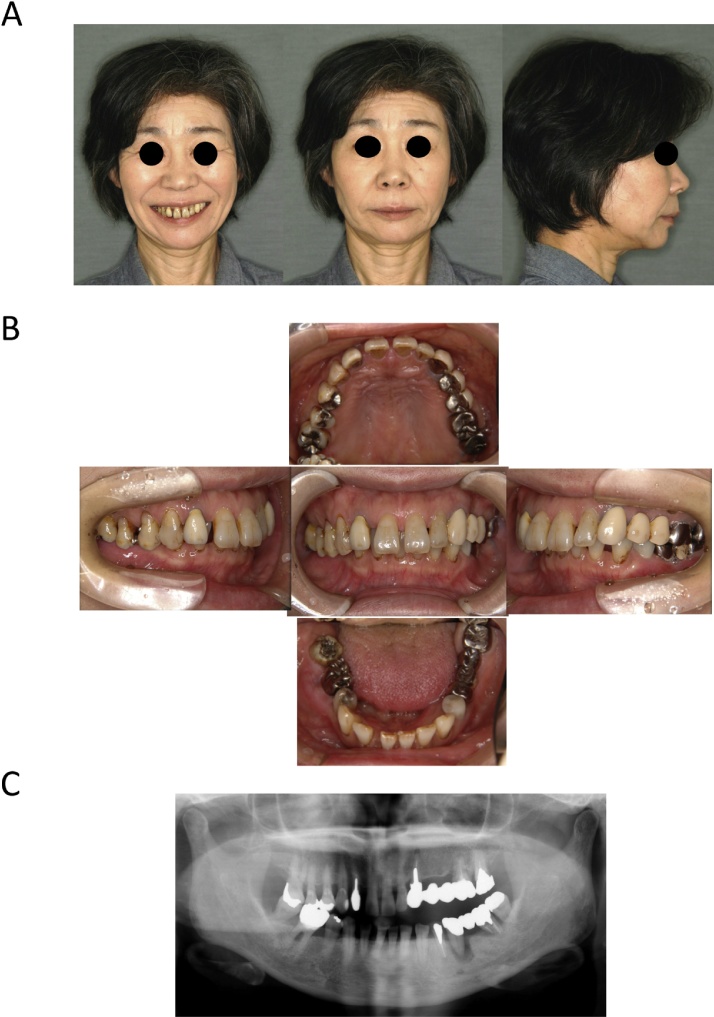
Extraoral and intraoral photographs and panoramic X-ray at pretreatment in Case 2.
